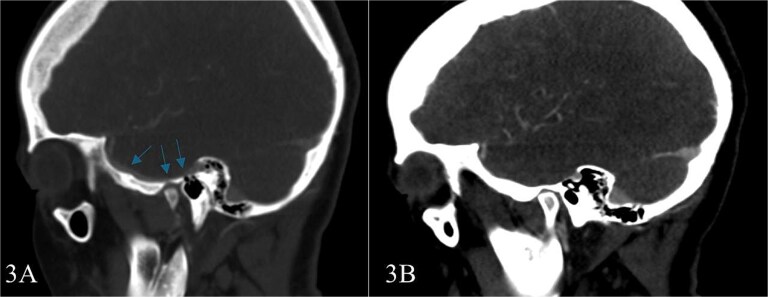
Consecutive images from late arterial phase CT scan reveals; the vascular nature of aforementioned observation which drain into dural venous sinus.
